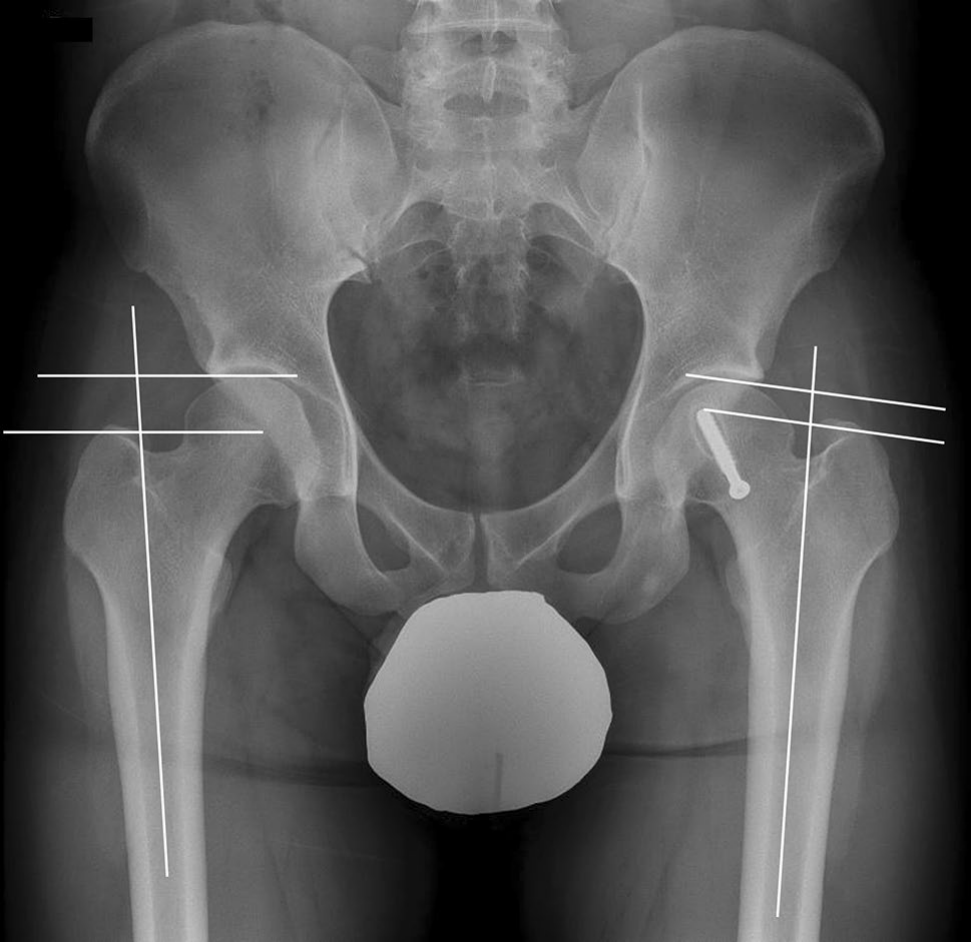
Measurement of the articulotrochanteric distance on supine anteroposterior radiograph of the hip. Articulotrochanteric distance difference was calculated as healthy side minus the involved side.
